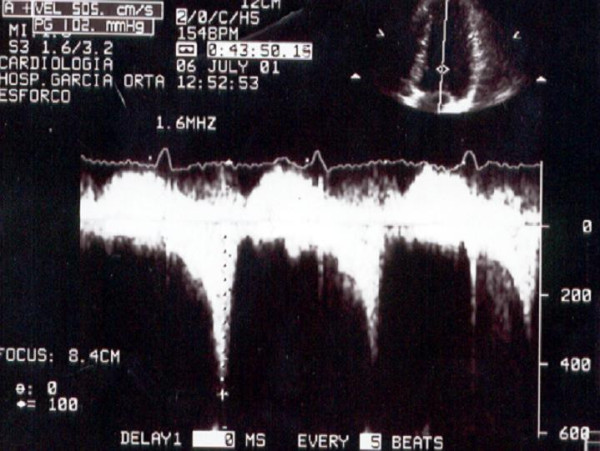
The flow obtained at that moment with continuous Doppler.
